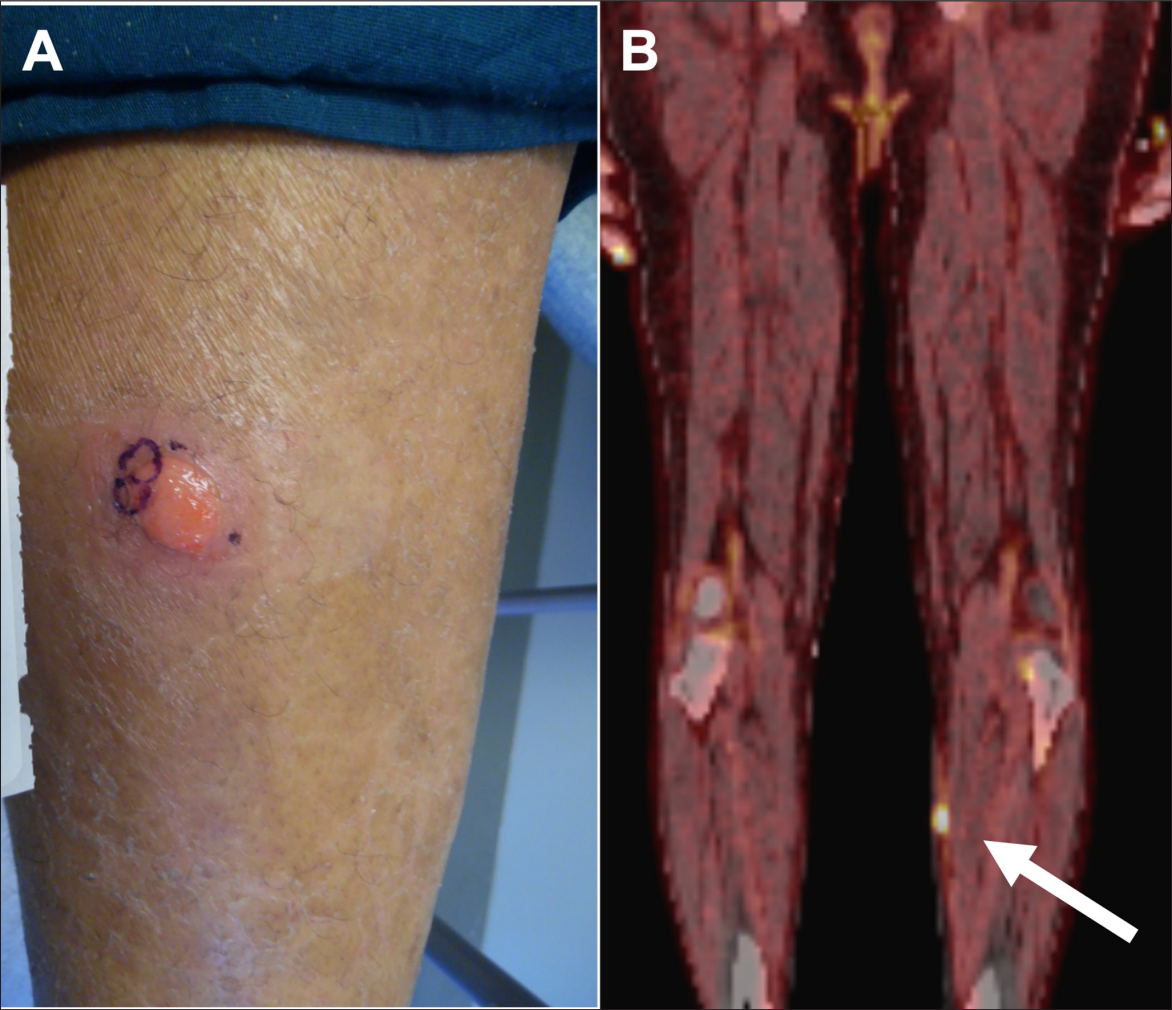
(A) Friable erythematous nodule of left medial leg. (B) Sagittal positron emission tomography with computed tomography with avid uptake in the same nodule of left leg (arrow).
